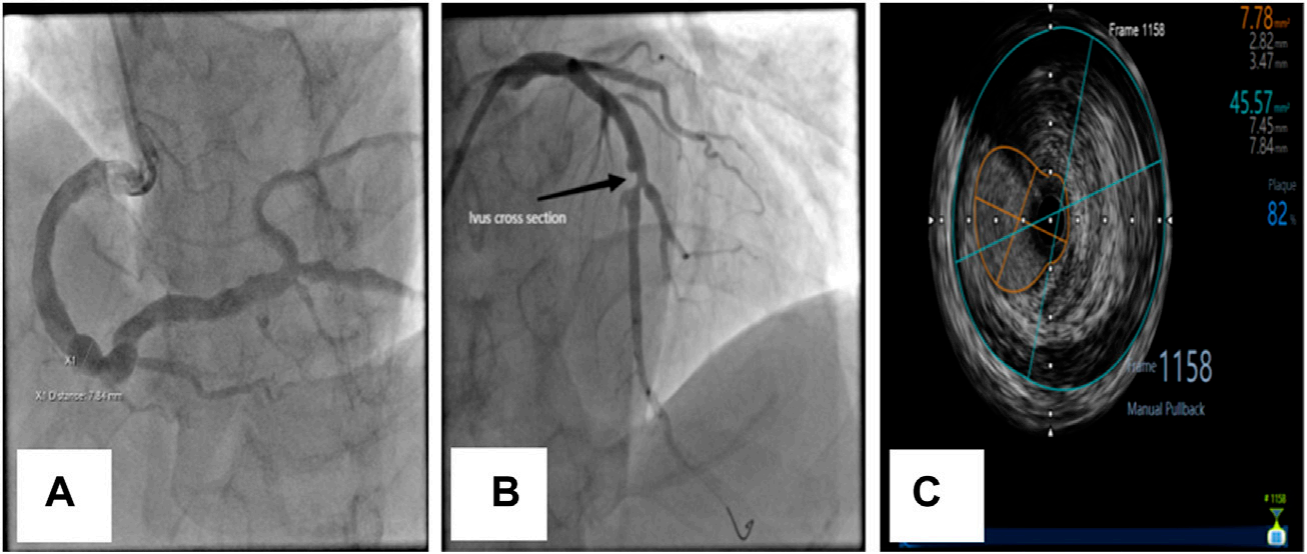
Coronary angiographic and intravascular ultrasonographic (ivus) images of CAE. (A) Diffuse dilation of the right coronary artery. (B) Diffuse dilation with significant atherosclerosis and stenosis of the left anterior descending (LAD) coronary artery in the same patient. (C) Ivus image of LAD.
